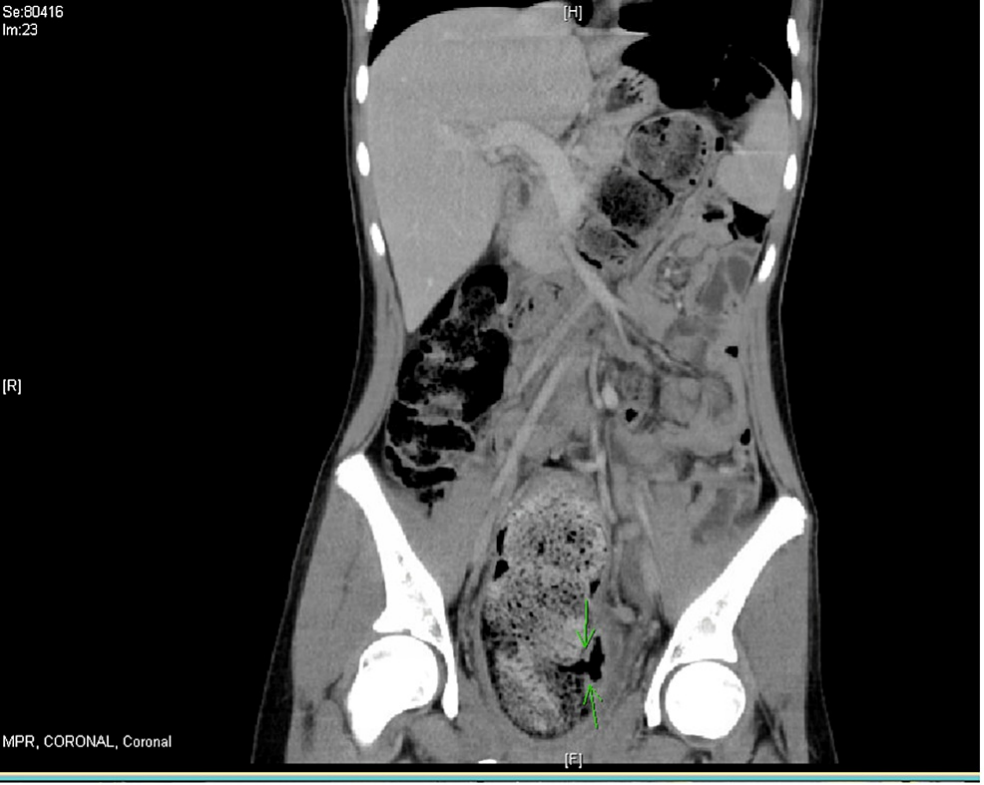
Coronal computed tomography imaging showing the site of rectal perforation (green arrows).
